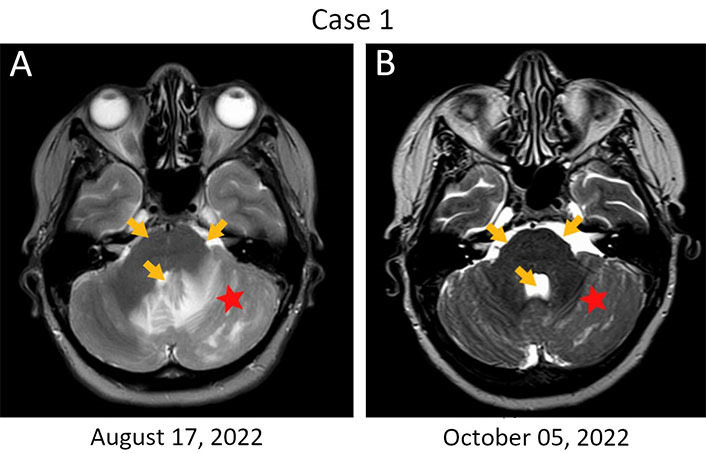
Rapid resolution of edema in a HER2-positive breast cancer patient with metastatic involvement of the brain. (A) Brain lesions at baseline. The first infusion of bevacizumab was given on September 6, 2022, when the patient was in coma; (B) MRI examination was performed on day 30 after the start of this treatment. Monotherapy with bevacizumab resulted in a significant reduction of the perifocal swelling in the left hemisphere of the cerebellum (red star); there is also a resolution of the compression of the pontine cistern and the fourth ventricle (orange arrows)
